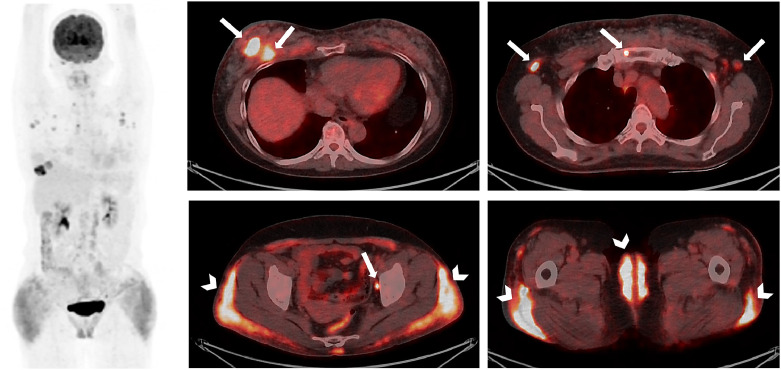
FDG-PET/CT demonstrated intense metabolic activity in 2 right breast malignancies (SUVmax 15.0, top left image, arrows) with metabolic activity in the bilateral axillary, mediastinal, para-aortic, and left pelvic lymph nodes (SUVmax 6.7) and sternal bony metastasis (SUVmax 7.6) (arrows). There was moderate metabolic activity in the bilateral subcutaneous gluteal foci, inguinal ligaments and bilateral labia majora (SUVmax 7.0), favored benign silicone-related inflammation (arrowheads, bottom right and left image).
